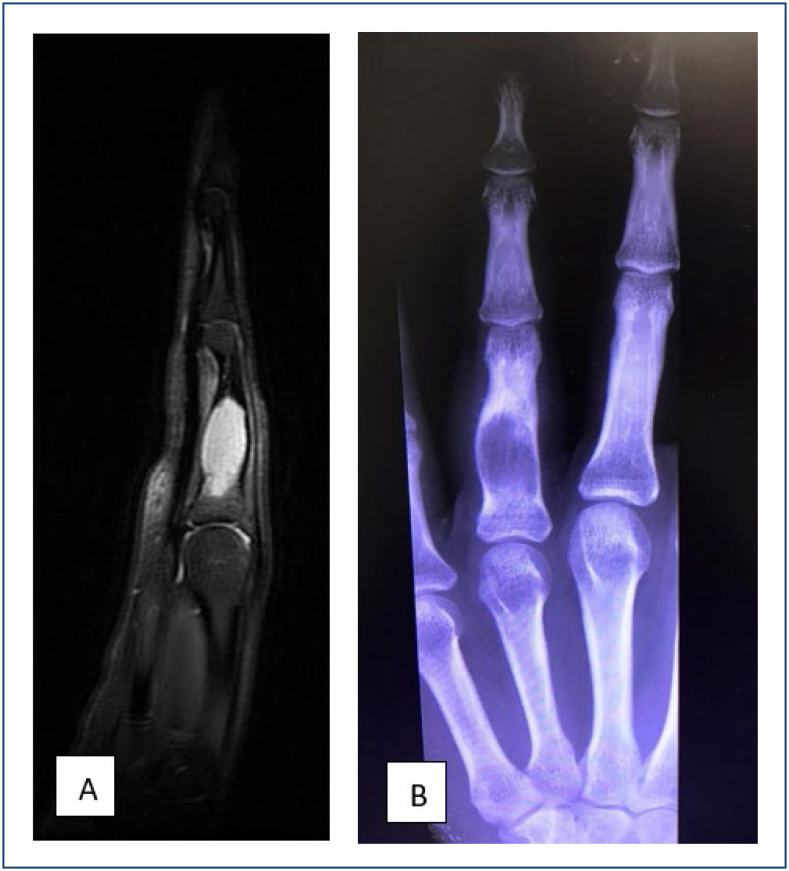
(A) T2-weighted magnetic resonance imaging, showing an enchondroma of the proximal phalanx of the left fourth digit, with high signal intensity of the lesion and cortical thinning. (B) X-ray AP view of the left hand showing a lytic, expanding lesion with cortical thinning in the proximal phalanx of the fourth finger, suggestive of enchondroma.
